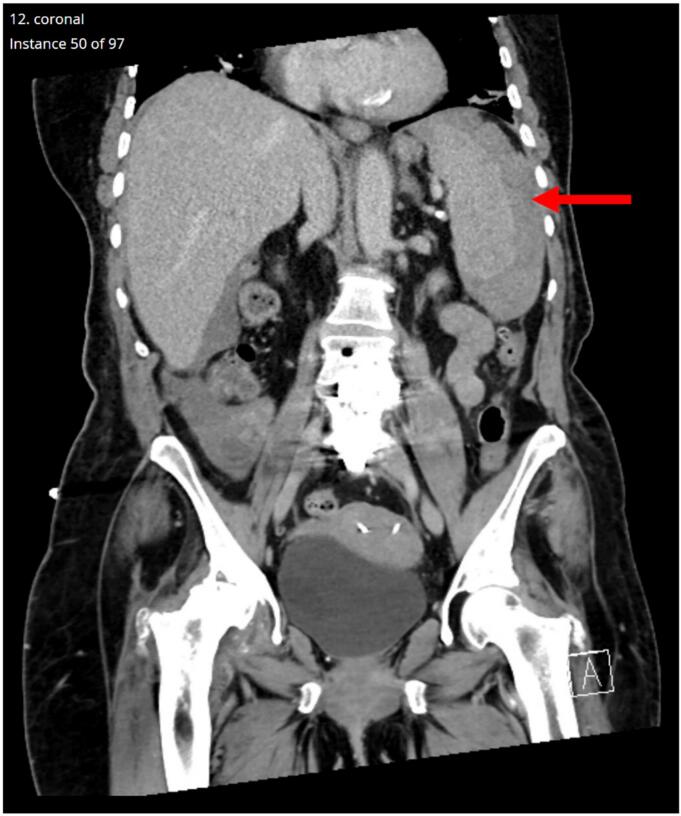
Coronal-view of contrast-assisted CT demonstrating acute splenic subcapsular hematoma (arrow).
